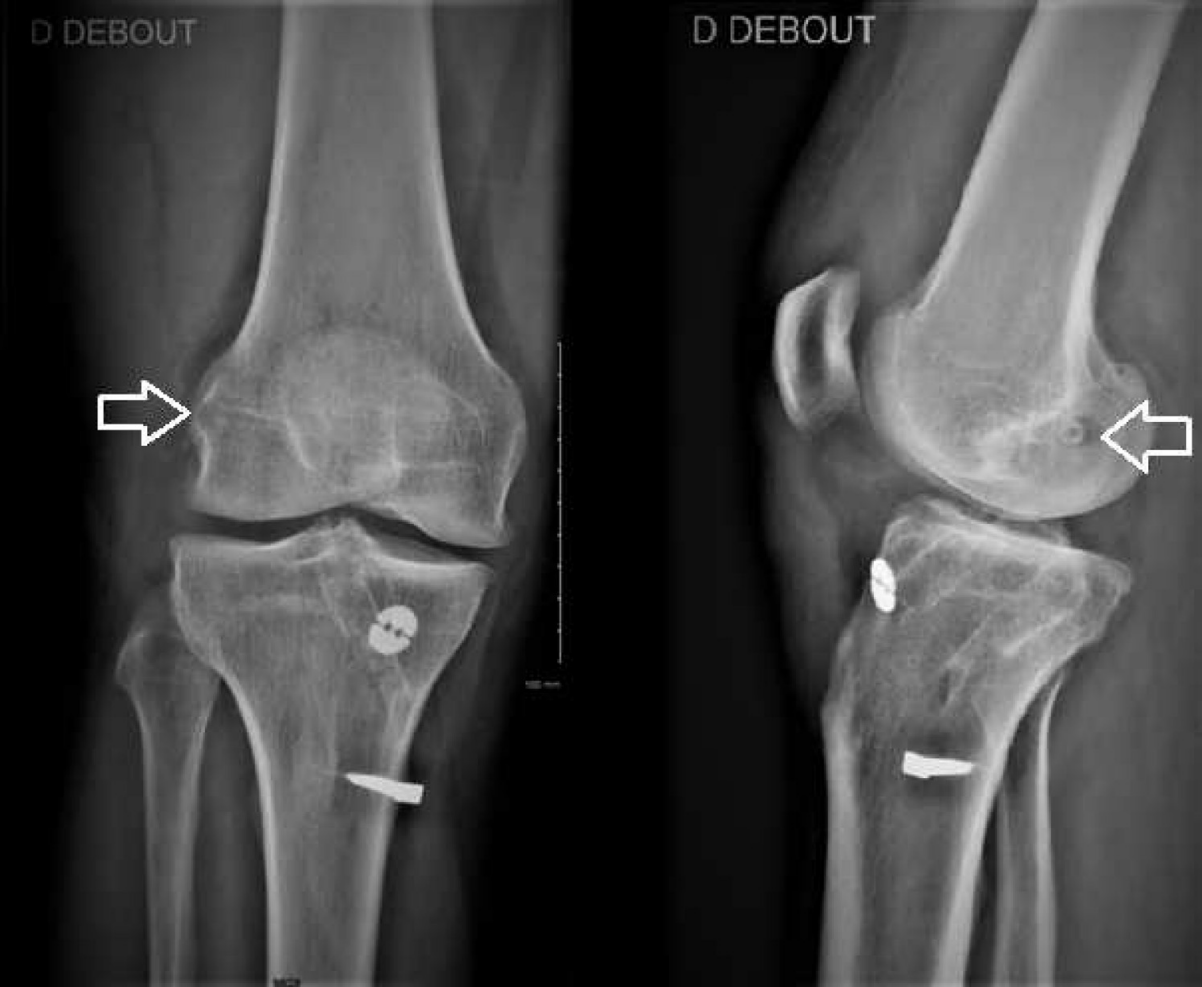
Postoperative radiographs of right (D) knee. We plan to drill the femoral tunnel with an outside-in technique, which minimizes the risk of tunnel overlap and, thereby, 2-stage revision. In this knee, we can see that the new femoral tunnel (arrows) is behind the lateral femoral condyle. There is no convergence between the existing and new femoral tunnels.
